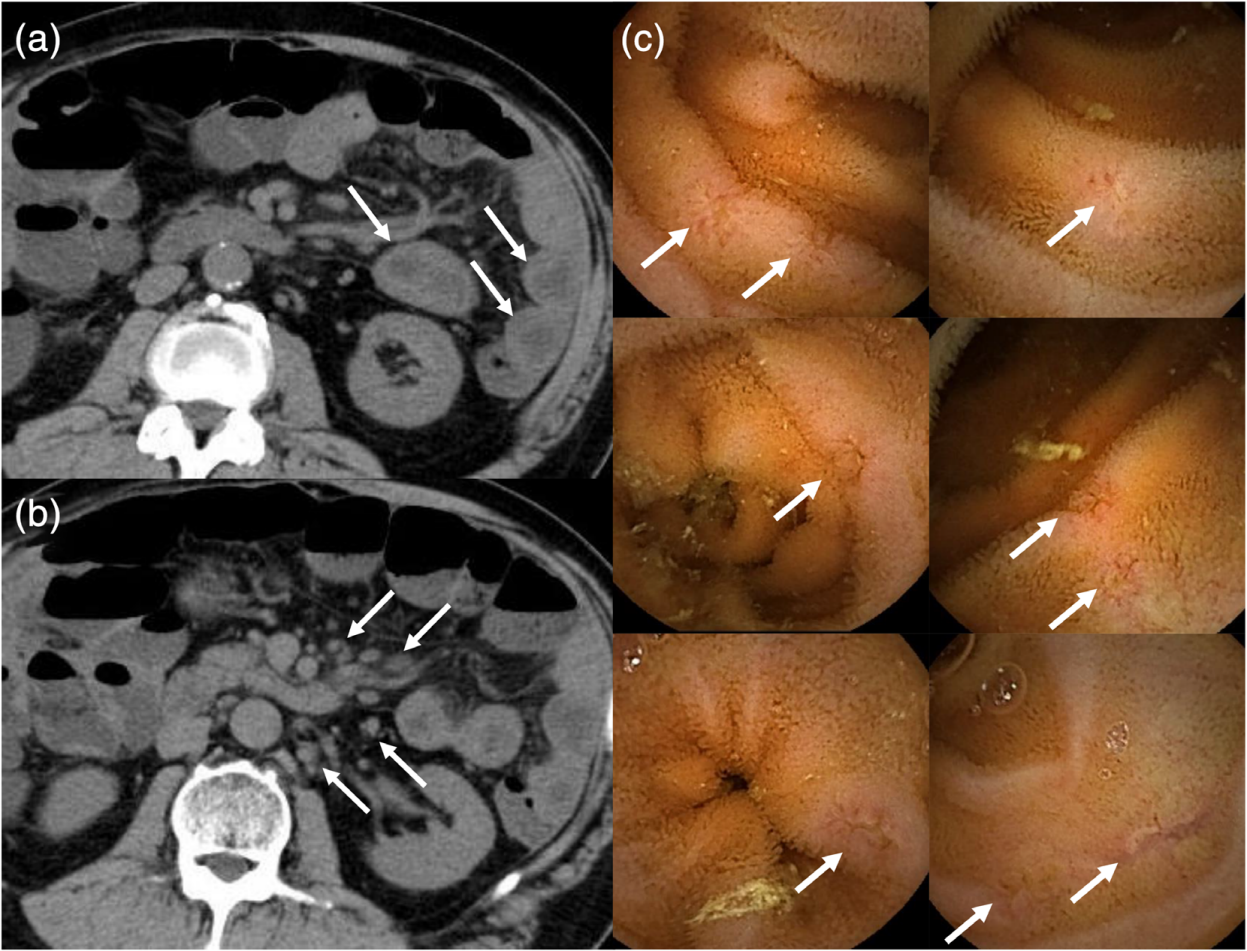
Computed tomography shows diffuse wall thickness of the jejunum (a, arrow) and lymph node swellings of the mesentery (b, arrow). Capsule endoscopy shows multiple erosions or small ulcers in the small intestine (c, arrow).
